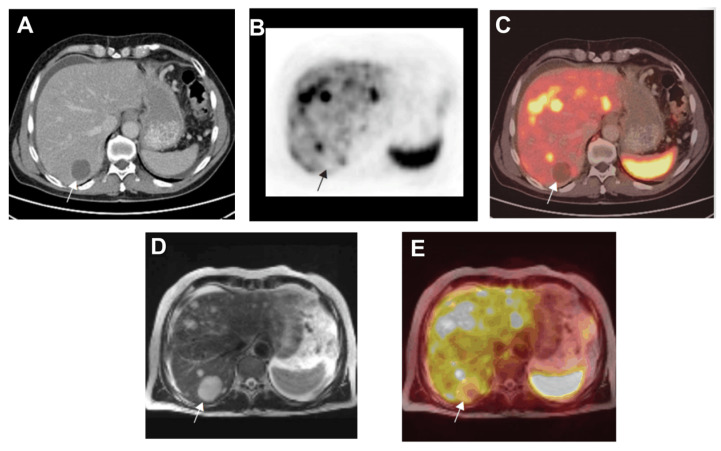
A patient with extensive metastatic disease of an intestinal carcinoid tumor. (A) Automatic fused image of PET/CT. (B) A venous-dominant contrastenhanced CT scan. (C) T2-weighted TSE image of MRI. (D) A manual fused image of PET/MRI. (E) with multiple hepatic metastases. PET, CT and the overall view of all MRI sequences identified a great cyst in the right lobe of the liver (arrow). Reprinted with the permission of [170].
